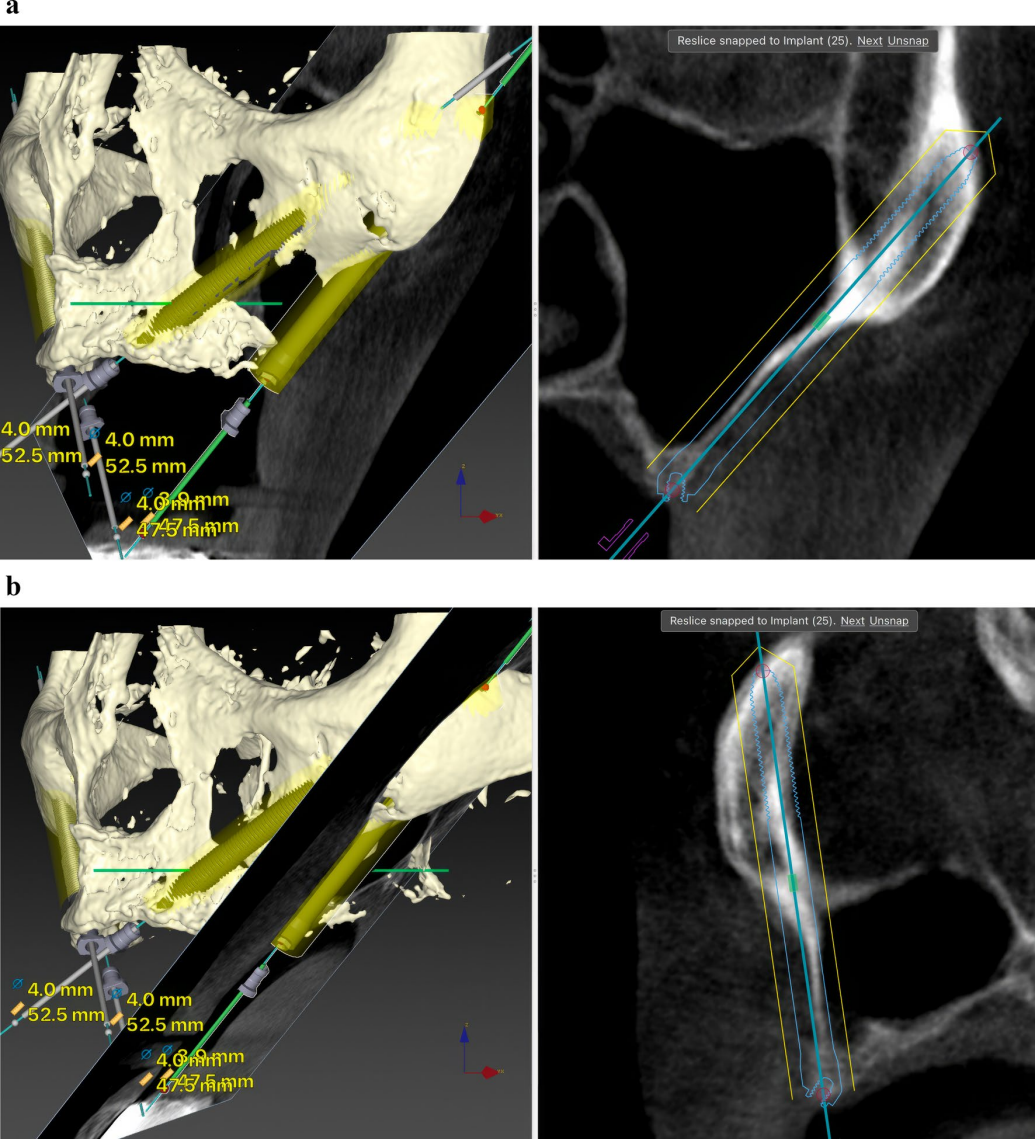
a Oblique CBCT slice, taken using DTX Studio Implant software, showing the planning and anatomy at the level of the second premolar/upper left first molar of the ZAGA Flat implant in Fig. 1. b The DTX Studio Implant software allows the rotation of the oblique plane attached to the implant. Thus, we can observe that the planning for the placement of the ZAGA Flat implant in Fig. 1 includes the partial use of the anterior wall of the temporal fossa. By increasing the number of cortices crossed by the implant, we will increase its primary stability
